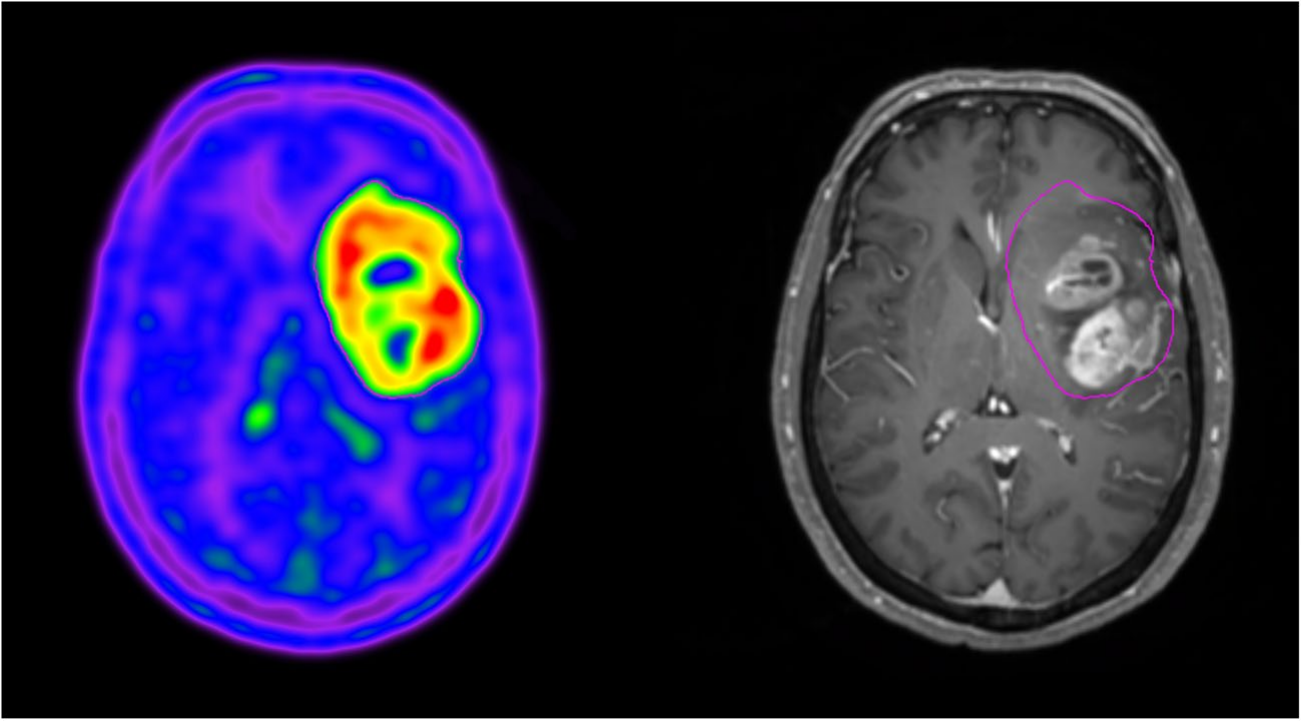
Transaxial ^11^C-acetate PET and contrast-enhanced T1-weighted MRI images in a patient with glioblastoma. Purple demarcation, ^11^C-acetate PET-based tumor margin. Intense ^11^C-acetate uptake is observed in glioblastoma and beyond the tumor boundary
